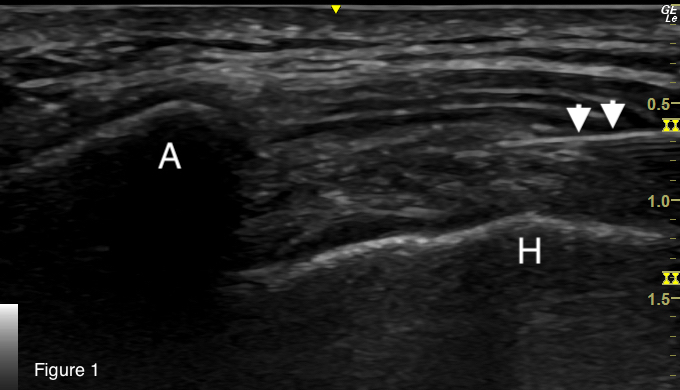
Ultrasound still image demonstrating ultrasound-guided approach to injection of the subacromial/subdeltoid bursa. (A: acromion; H: humeral head; arrowheads: needle.)
